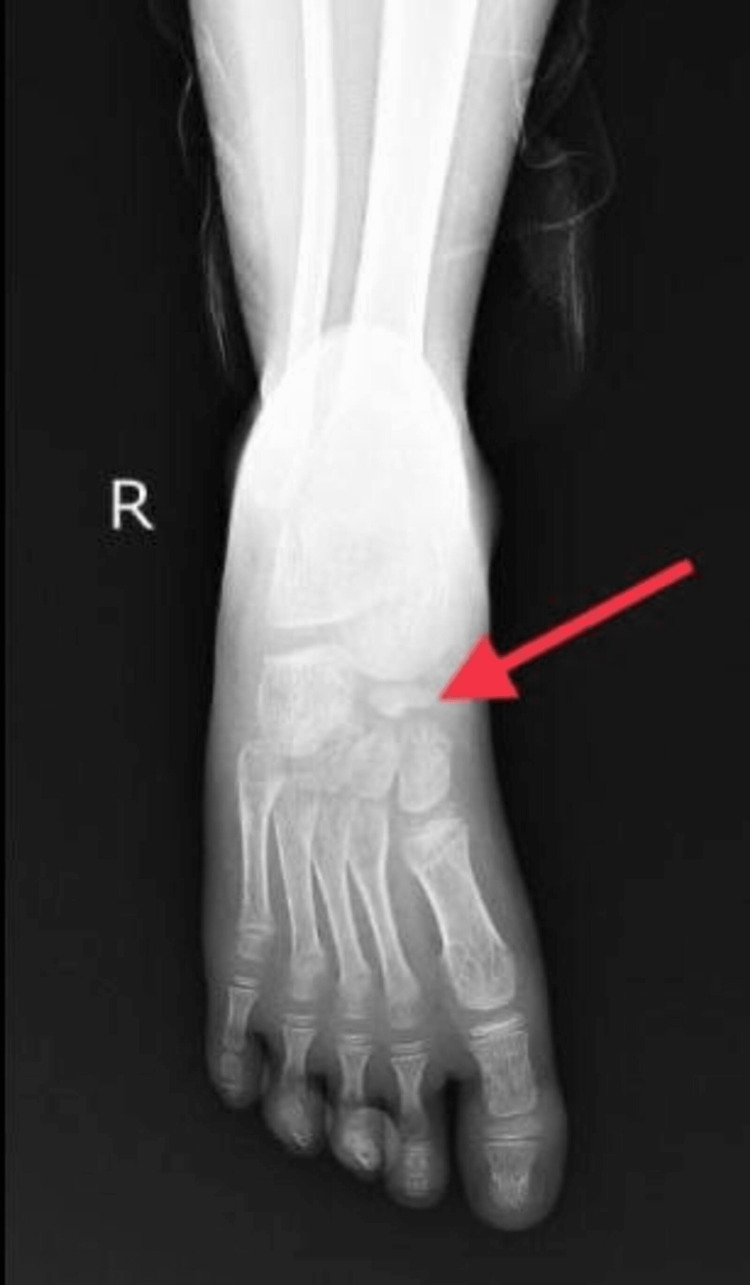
X-ray anteroposterior view of the right foot and ankle
